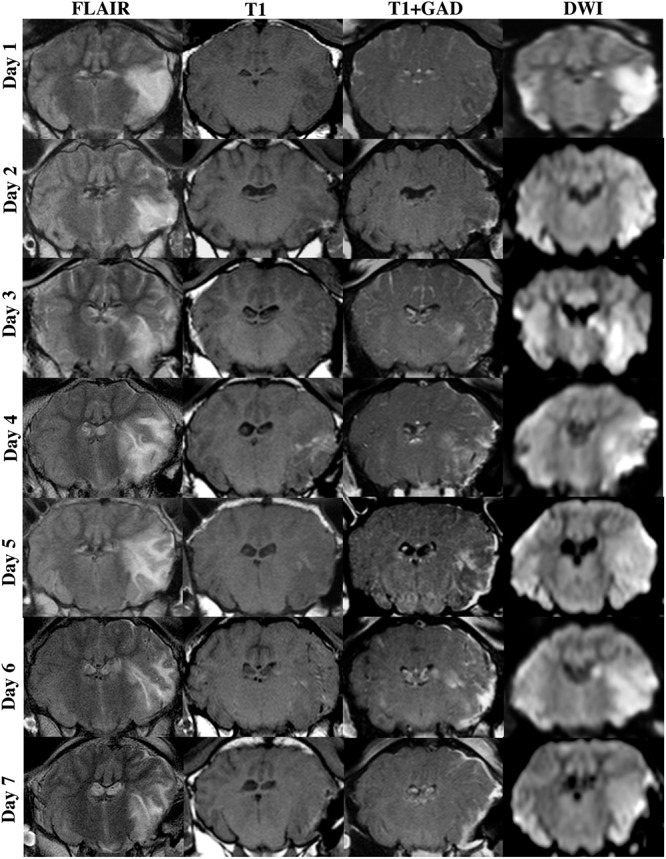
MRI findings following transient MCAO. Cerebral edema, as shown in coronal FLAIR MRI images, evolved from 1–6 days following stroke, beginning to resolve by 7 days. This evolution in cerebral edema was associated with enhanced extravasation of GAD across the BBB as seen on T1 weighted post-contrast series (T1+GAD) when compared to pre-contrast T1 series. Lesion volume, as shown in DWI MRI images, was comparable across time-points following stroke.
